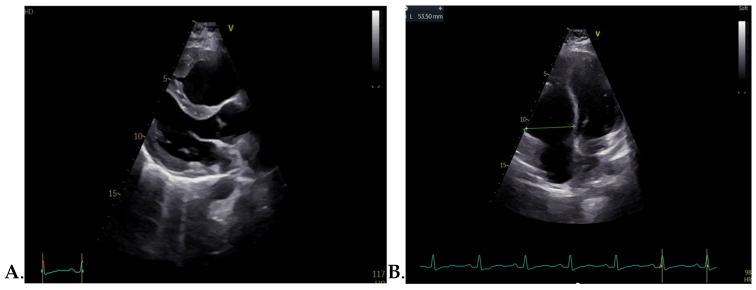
Transthoracic echocardiography at admission demonstrating marked right ventricle dilatation (A) Parasternal shorts axis view (B) Apical four chamber view.
